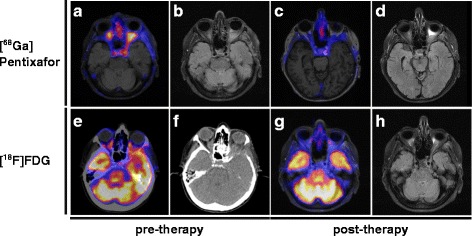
Response assessment with CXCR4-directed PET imaging compared to standard [^18^F]FDG PET. a, c, g PET/MR fusion. b, d, h MR imaging. e PET/CT fusion. f CT imaging
